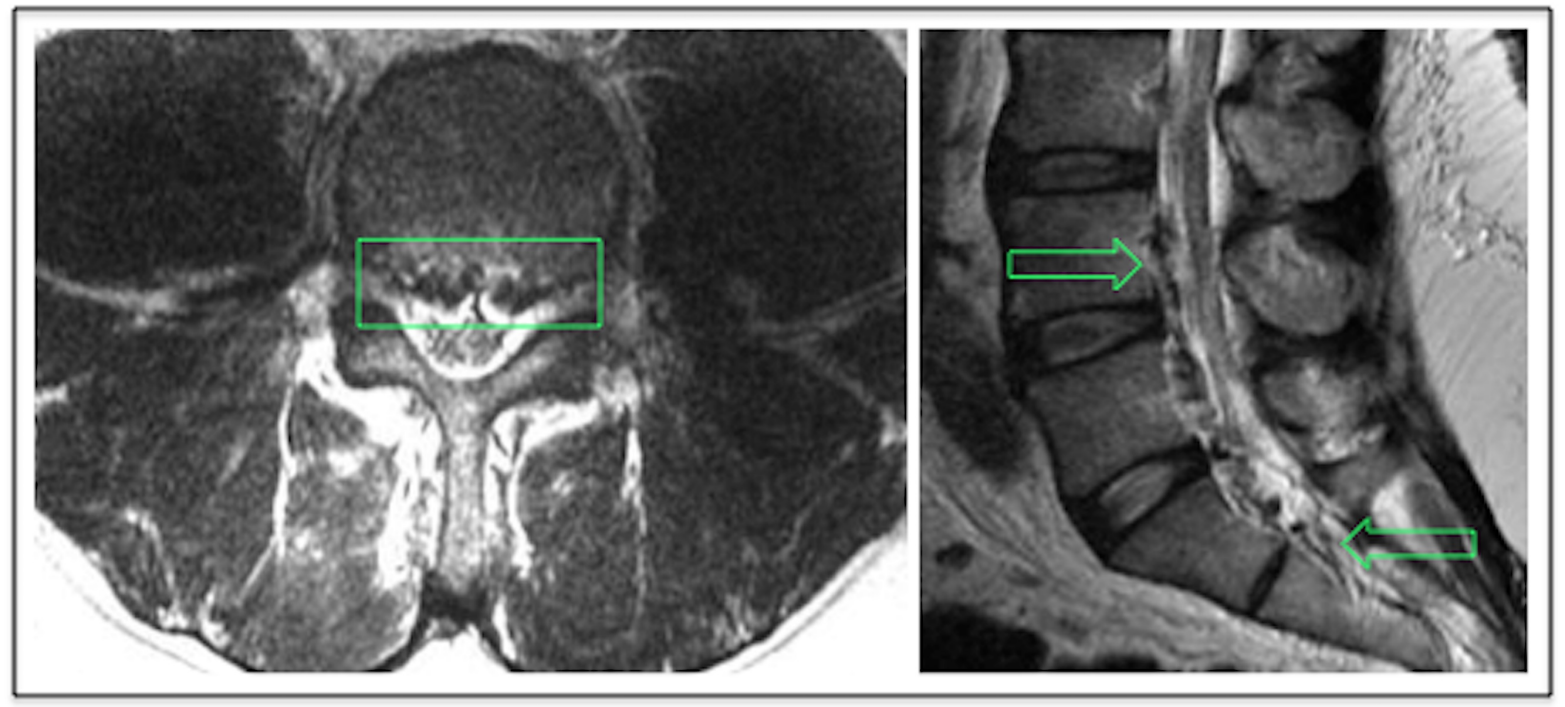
Postoperative lumbar MR imaging The left pane displays the MRI T2 axial section showing prominent flow voids (boxed) at the level of L4. The right pane displays an MRI T2 sagittal section with prominent ventral epidural enhancement (arrows) that is consistent with engorged vessels. This is shown causing narrowing of the thecal sac. This effect is most severe at the L4 vertebral level (boxed region of left image).
